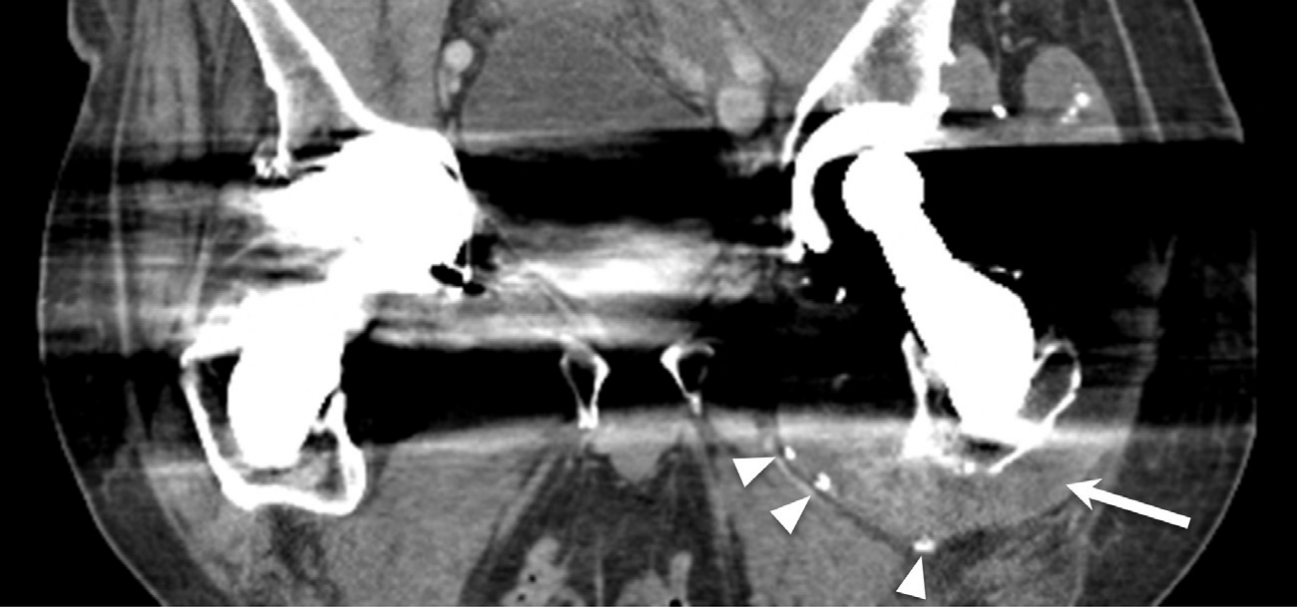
Coronal CT image of the pelvis with soft tissue windows demonstrates abnormal soft tissue attenuation around the left total hip arthroplasty (arrow). Evaluation is limited by streak artifact from the hardware. There are foci of metallic debris around the left hip (arrowheads).
